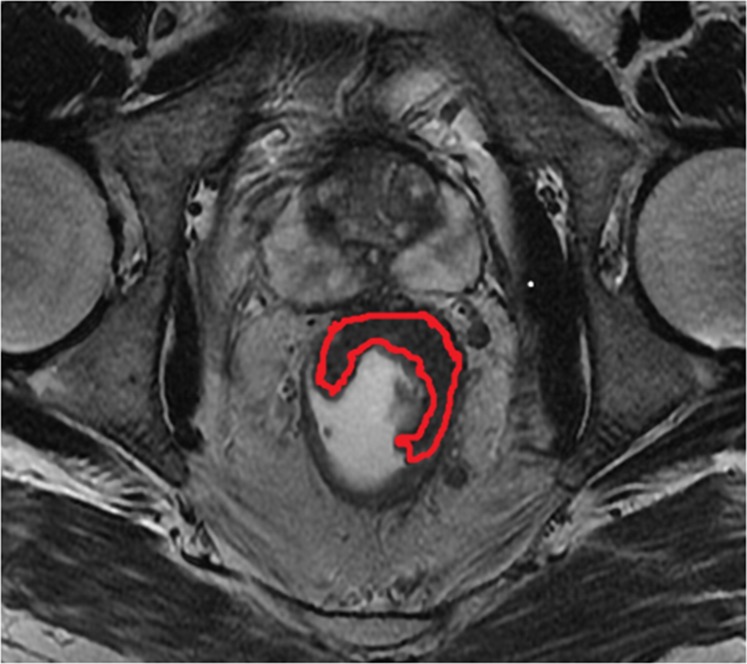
The region delineated by red curved line indicates indicates the measurement of maximum tumor area(MTA) on the oblique axial T2-weighted imaging
